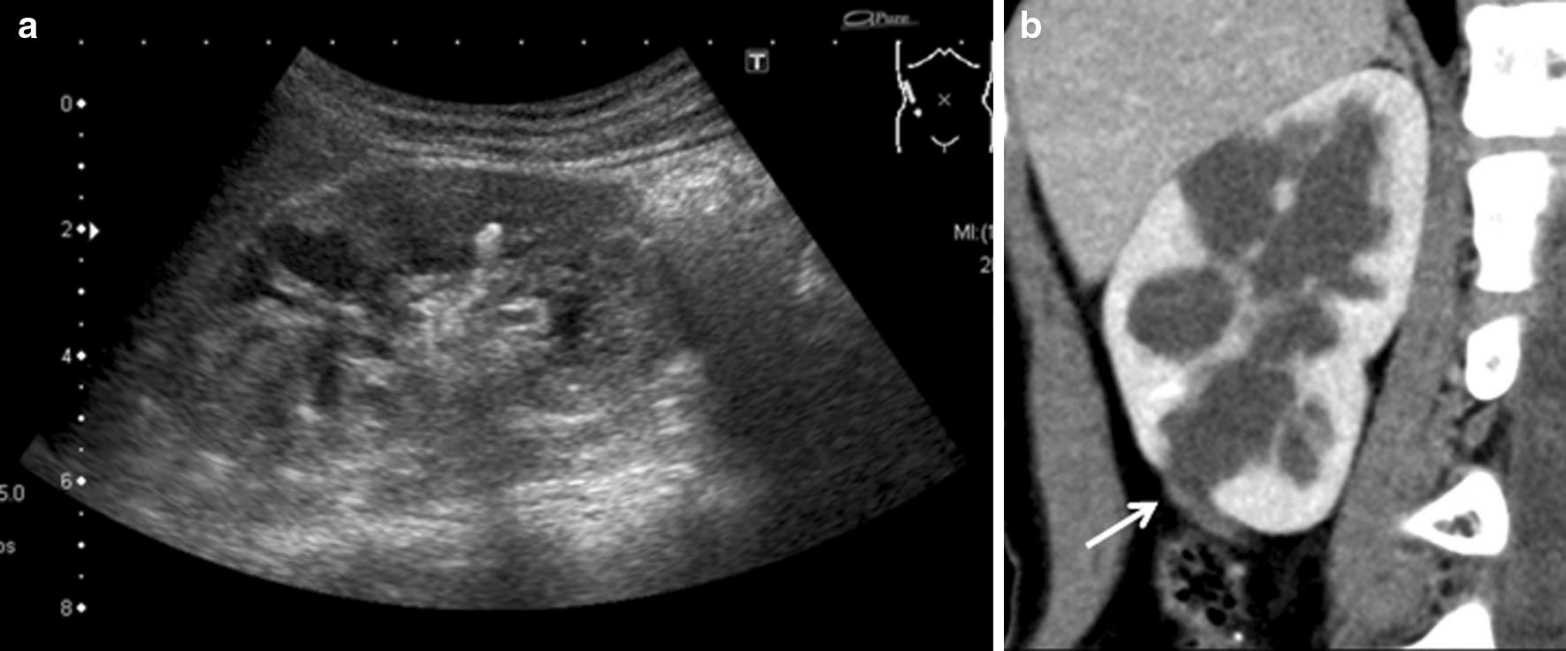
a Postoperative US showed improvement in the hydronephrosis 6 months after surgery. b CT scan revealed patency of the anastomosis (*white arrow*) at 15 months postoperatively
